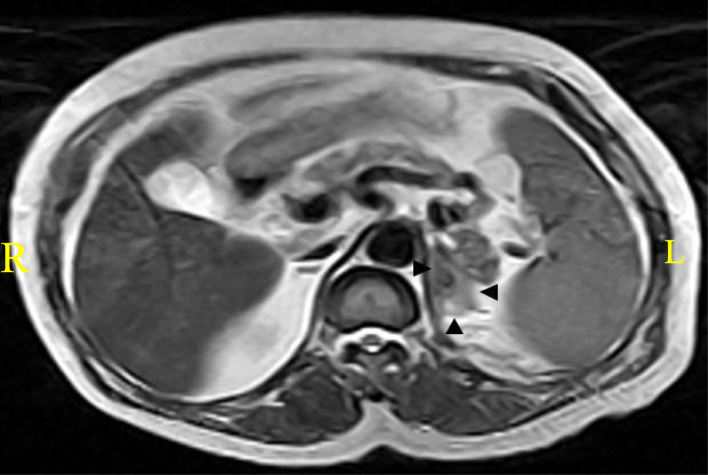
Axial T2WI MRI of the abdomen showing micro-lobulated soft tissue lesion arising from left suprarenal gland (arrow heads) displaying heterogeneous SI at T2WI
